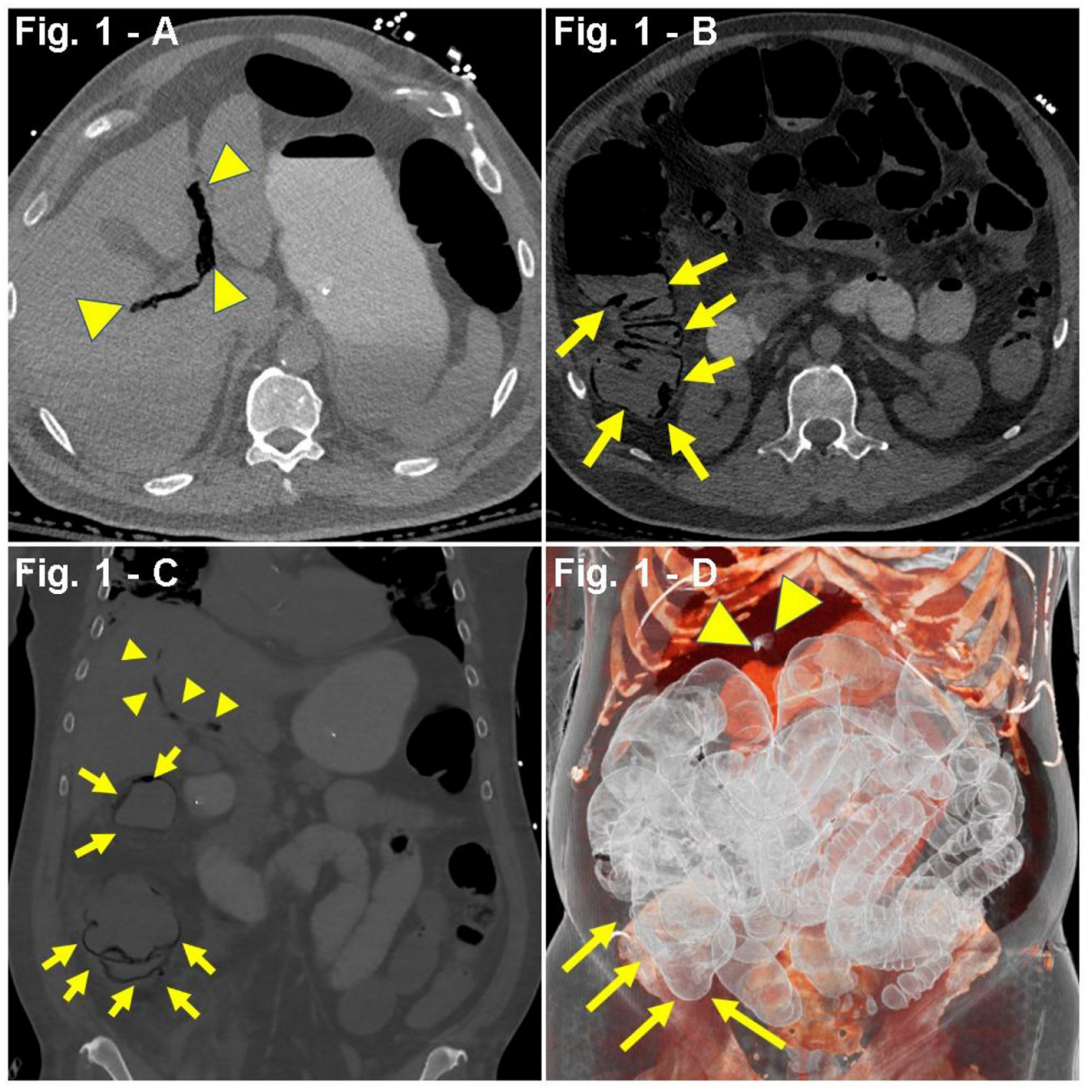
(A–D) Case presentation of 65-year-old male patient with COVID-19, 5 days after tocilizumab (TCZ), non-contrast abdominal CT. (A,B) Axial, (C) coronal, and (D) 3D reconstruction, pneumatosis intestinalis (PI) involving ascending colon (yellow arrows), with dilated multiple right lower quadrant small bowel loops with mesenteric and portal venous gas (yellow arrowheads).
